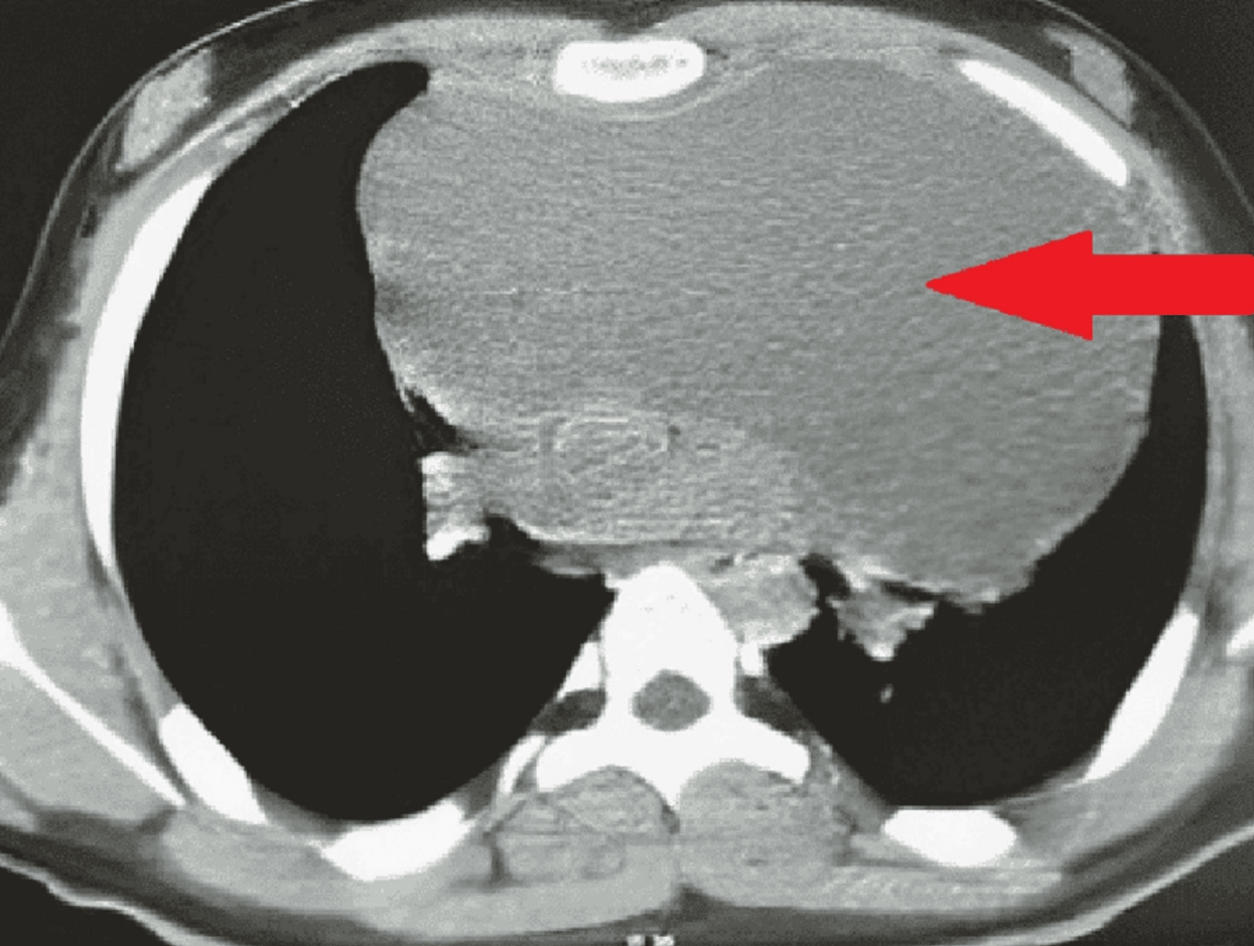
High-resolution resolution computed tomography image of anterior mediastinal well-differentiated cystic mass
